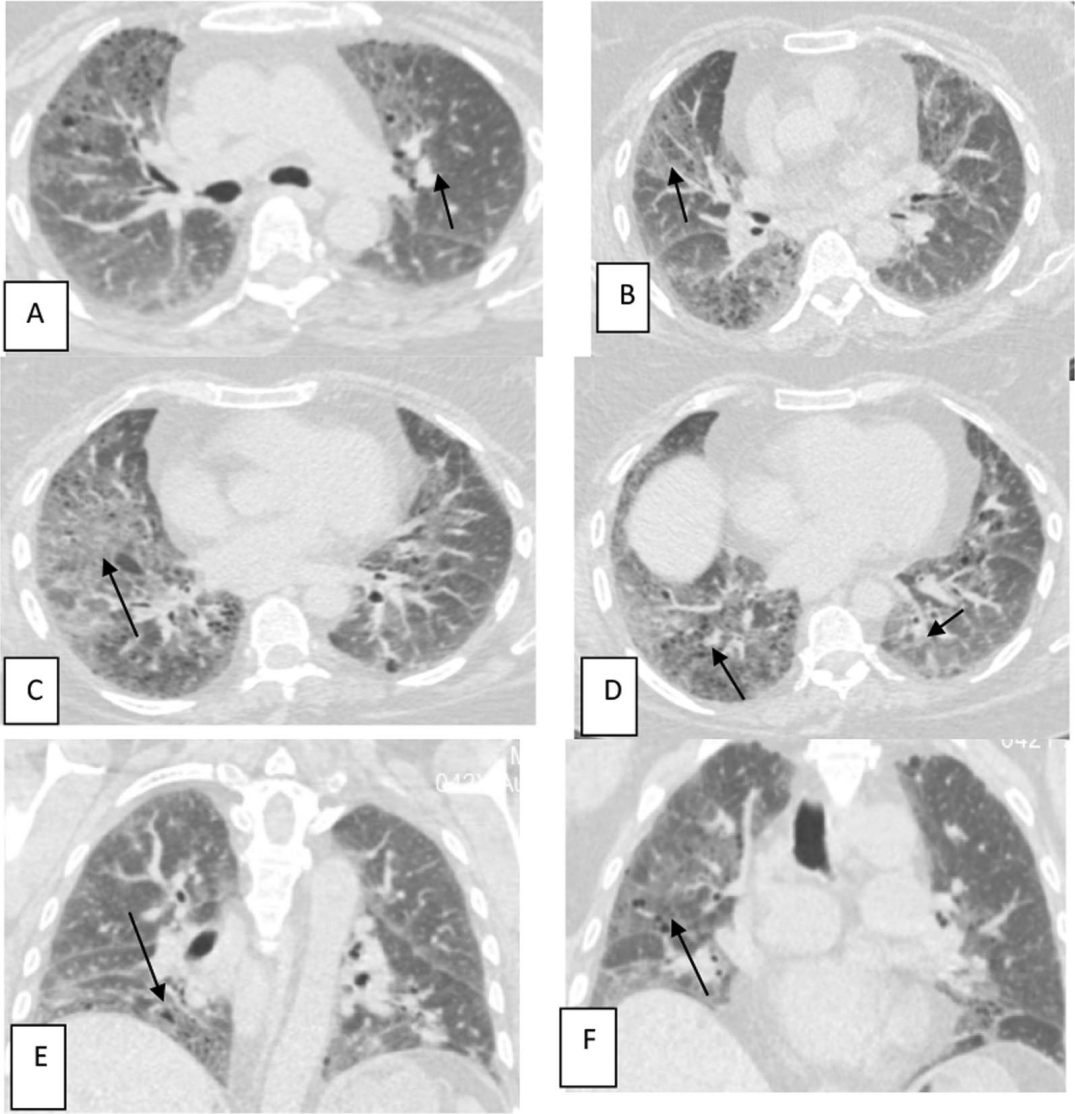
Female patient aged 35 years old, with history of COVID-19 infection 3 month ago, presented by dyspnea on exertion and tachypnea. Axial (A–D) and coronal (E, F) CT images show multiple patchy areas of ground-glass density with reticular infiltration and interlobular septal thickening, associated with multiple tiny cysts (< 1 cm) (black arrows); with lower lobes predominance, these changes are consistent with post-COVID-19 interstitial lung fibrosis
